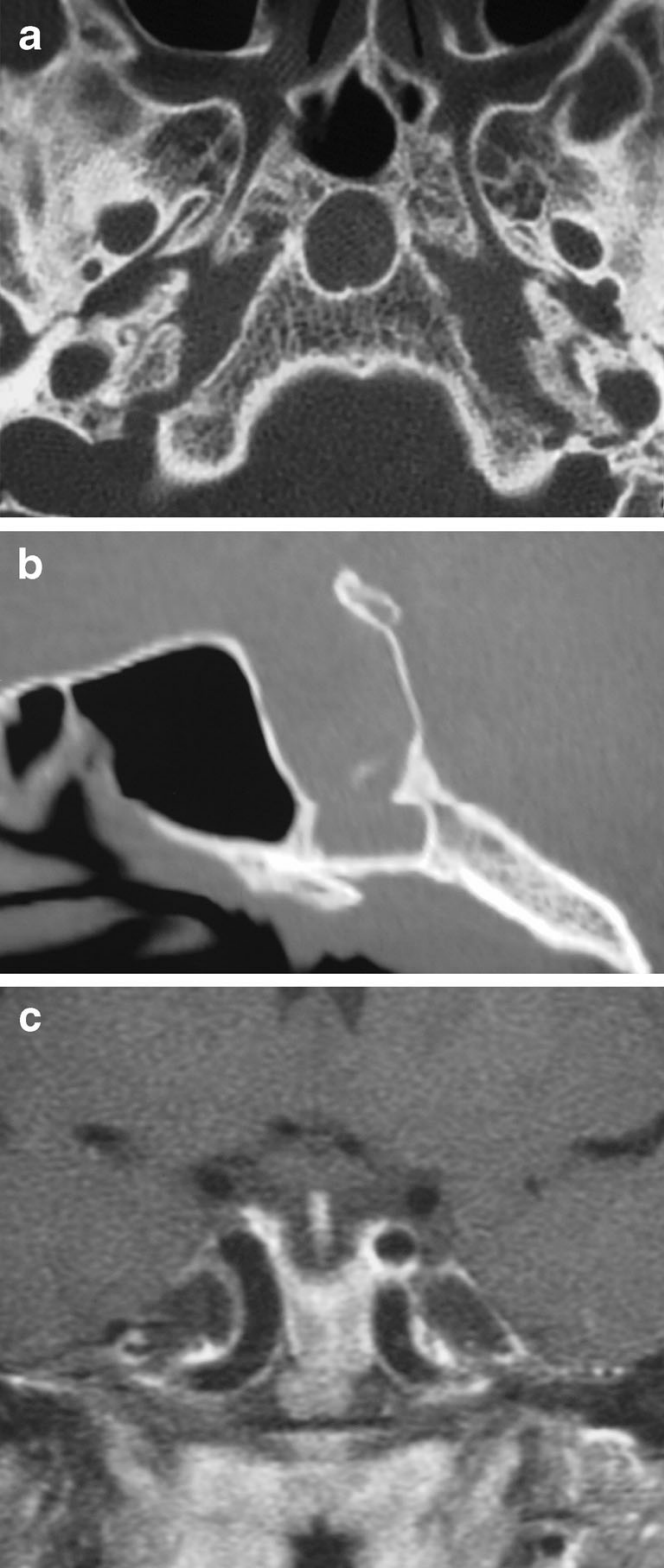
Persistent craniopharyngeal canal. Axial (a) and sagittal (b) CT shows a markedly enlarged persistent craniopharyngeal canal. Coronal fat-suppressed T1WI shows inferior extension of enhancing pituitary tissue within the abnormally enlarged craniopharyngeal canal (c)
